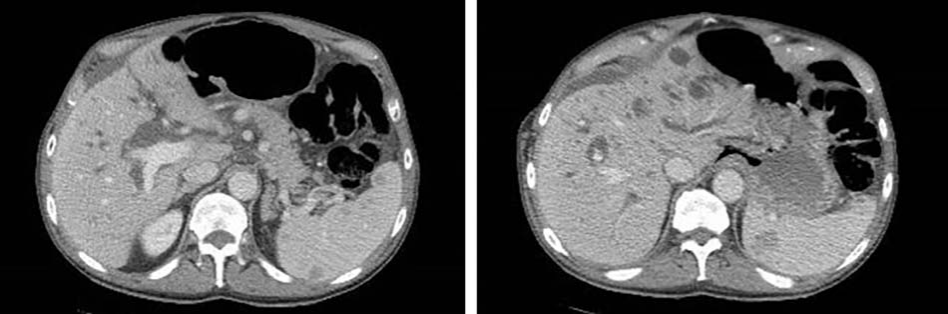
CT of the abdomen and pelvis demonstrating severe intrahepatic and extrahepatic biliary ductal dilation and multiple splenic hemangiomas.
